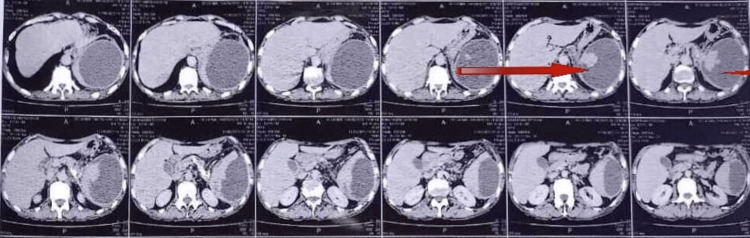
CECT of the abdomen axial section showing an atrophic pancreas and splenic abscess CECT - contrast-enhanced computed tomography
